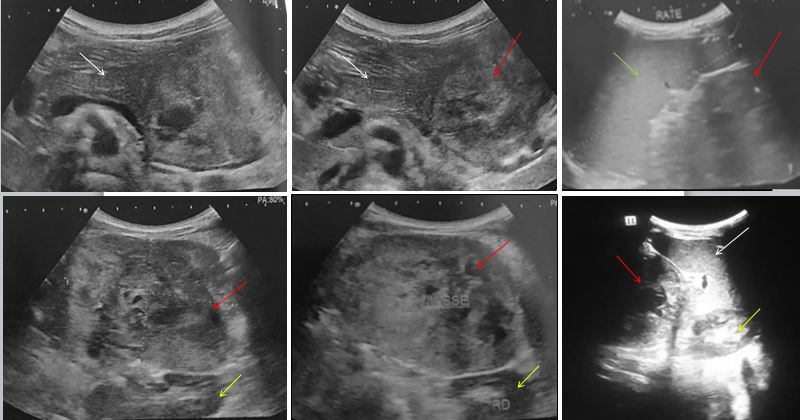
Ultrasound revealed a subcostal solid mass located on the left hypochondrium (red arrow), having a contact with the tail of the pancreas (white arrow) and the spleen (green arrow). It was hypoechoic, heterogenous. The left kidney was pushed back without being involved (yellow arrow)
